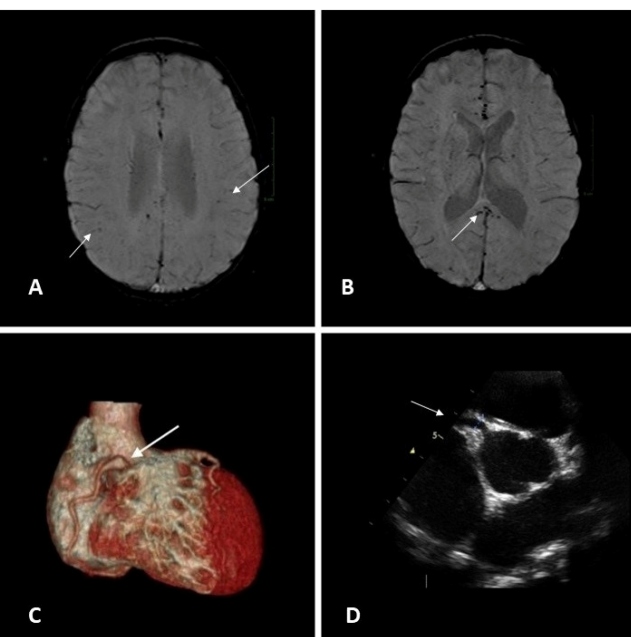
Brain resonance showing multiple areas of hypointense signal in the subcortical white matter of the cerebral hemispheres (A) and in the splenium of the corpus callosum (B). The arrow in the coronary angiotomography points to the ectasia region of the right coronary (C). Echocardiogram showing ectasia of the coronary artery (D).
